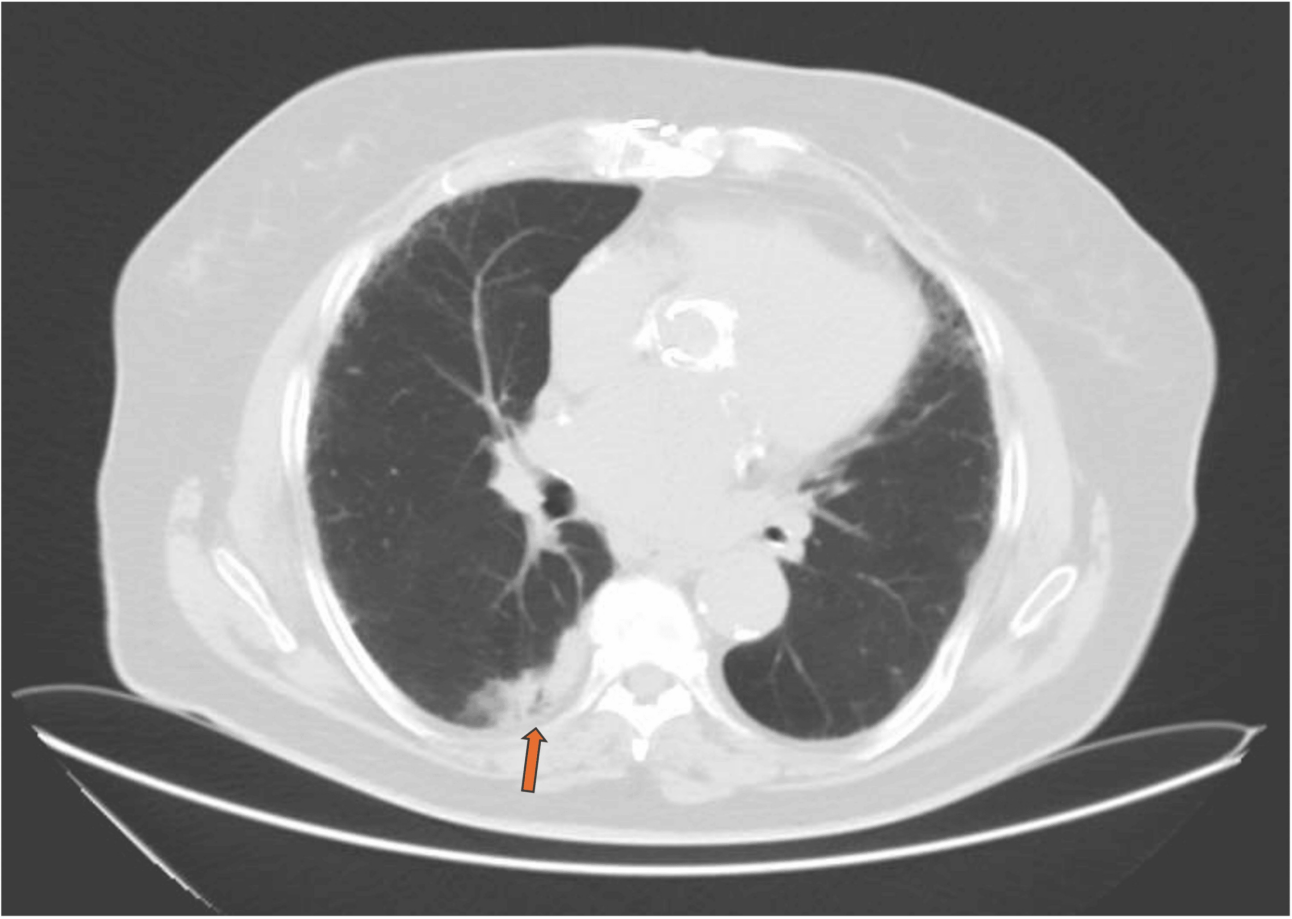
Chest CT scan showing a heterogeneous condensation in the posterior aspect of the lower right lobe (orange arrow)
